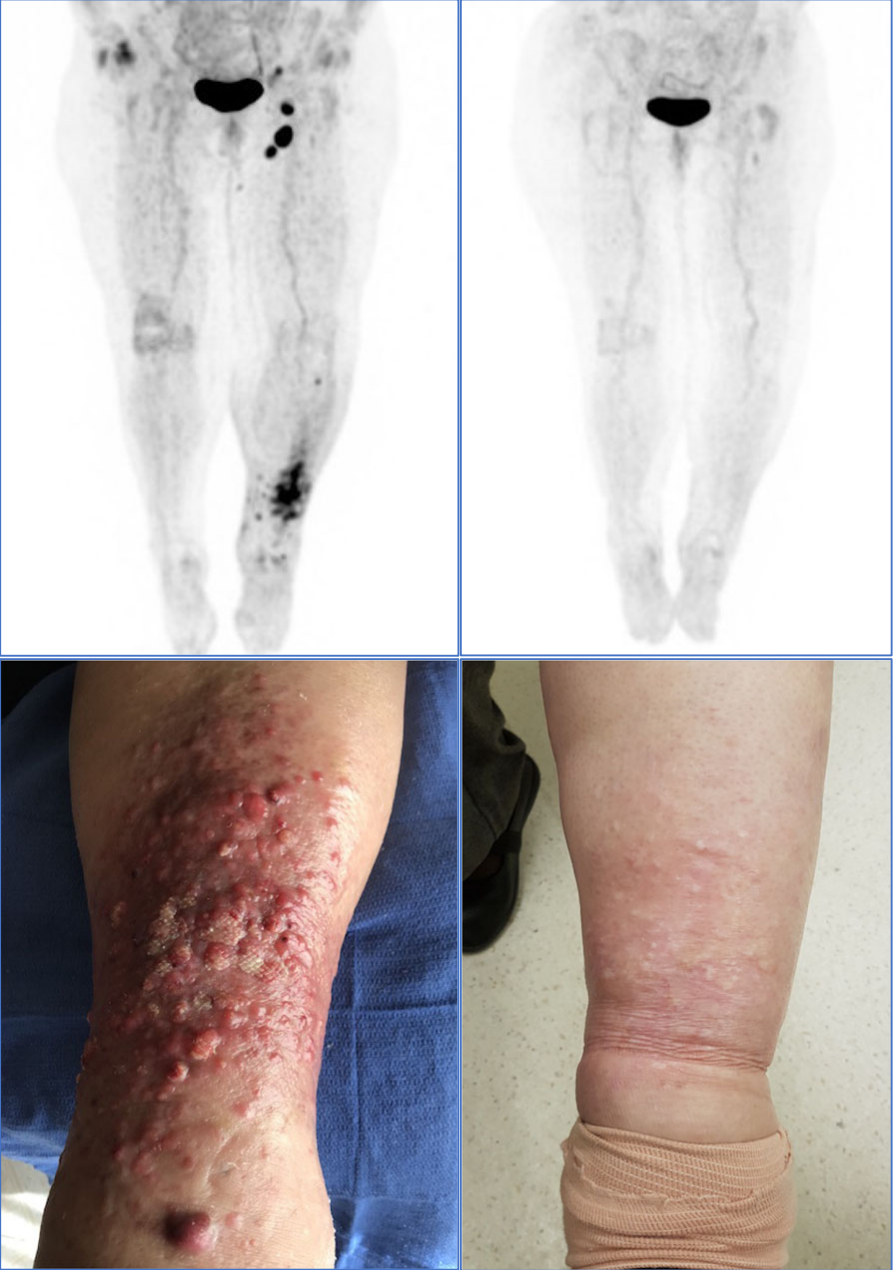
FDG-PET and Clinical Response. FDG-PET maximum intensity projection in July 2018, prior to pembrolizumab treatment (top left panel) and in April 2019, over 6 months after her fourth and final pembrolizumab treatment (top right), with corresponding clinical photography of the patient's left shin from these time points (bottom left and bottom right respectively). In 2018, lesions over left shin were erythematous non-tender papules and nodules, with biopsy confirming metastatic melanoma
